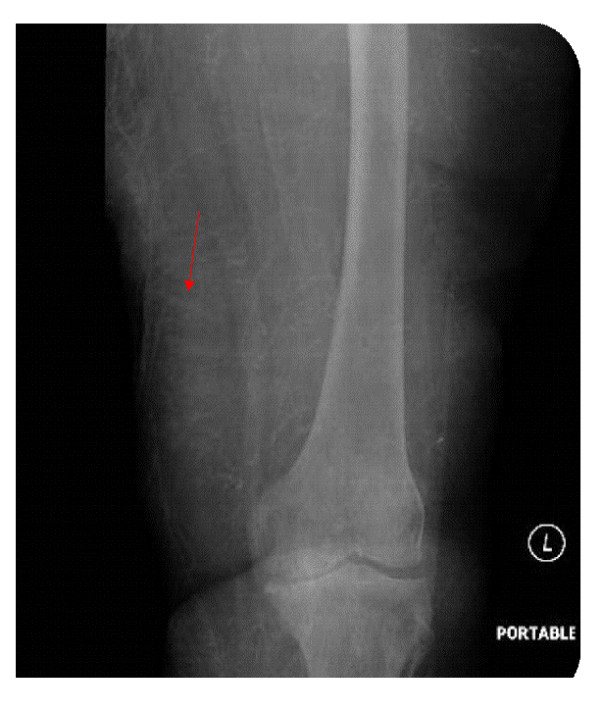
X-ray of left thigh (AP view) showing extensive vascular Calcification.
